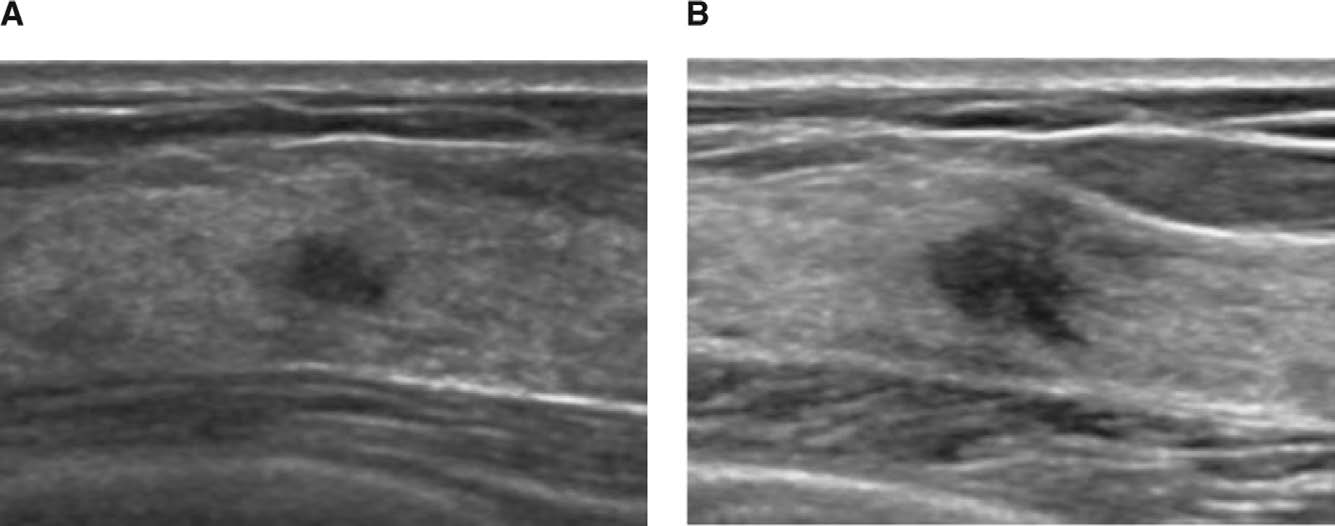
Screening US of a 50-yr-old woman. (A) Prior US shows 0.5 cm sized hypoechoic mass with an irregular shape and indistinct margin. This mass was initially assessed as BI-RADS category 3 but upgraded to BI-RADS category 4 in reassessment due to its shape and margin. (B) A 7-mo follow-up US reveals a size increment of the mass to 0.8 cm. This mass was confirmed as invasive ductal carcinoma after surgery. BI-RADS = Breast Imaging Reporting and Data System, US = ultrasound.
